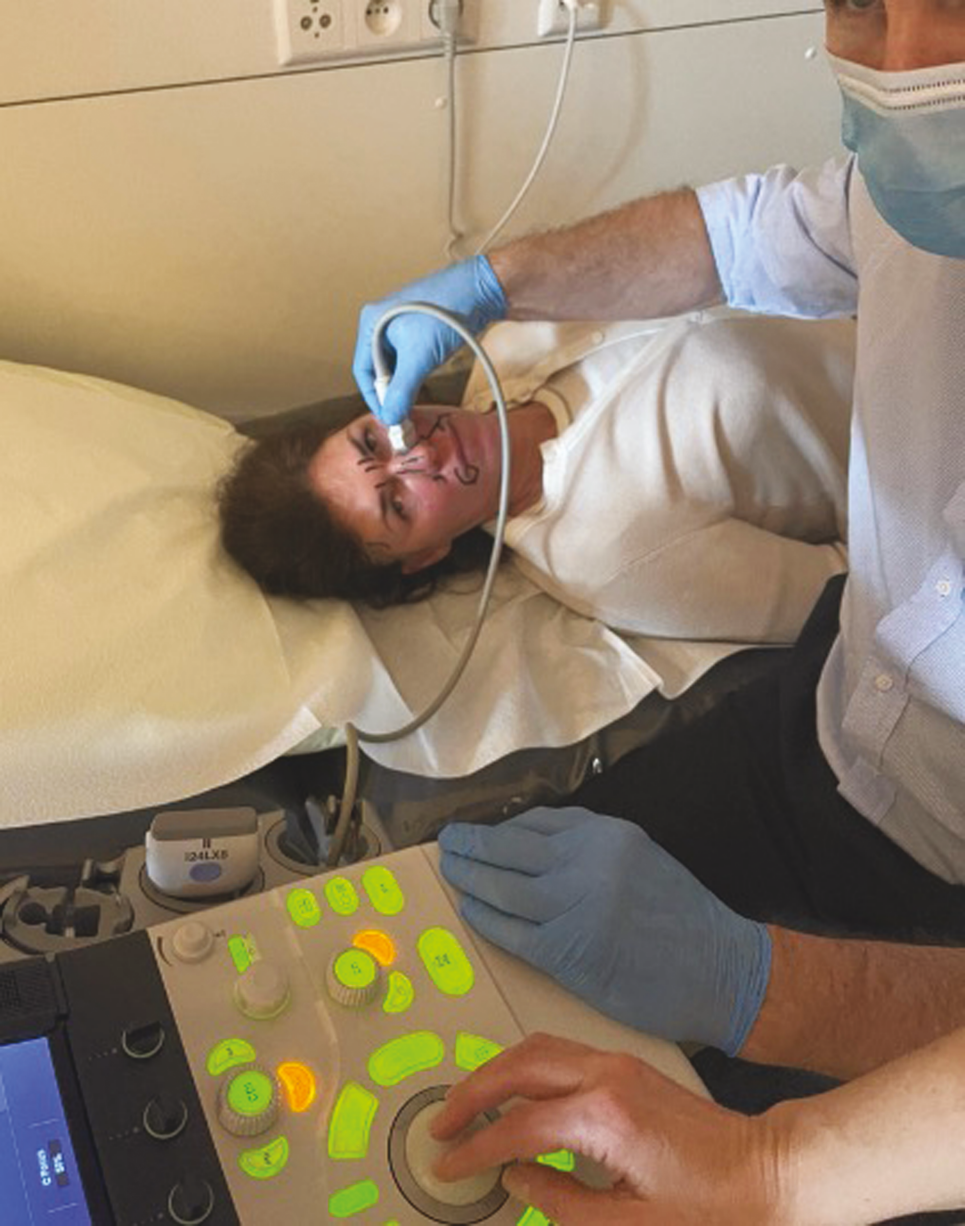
Determining the exact location of a superficial artery of the face using duplex ultrasound on a 39-year-old female.
